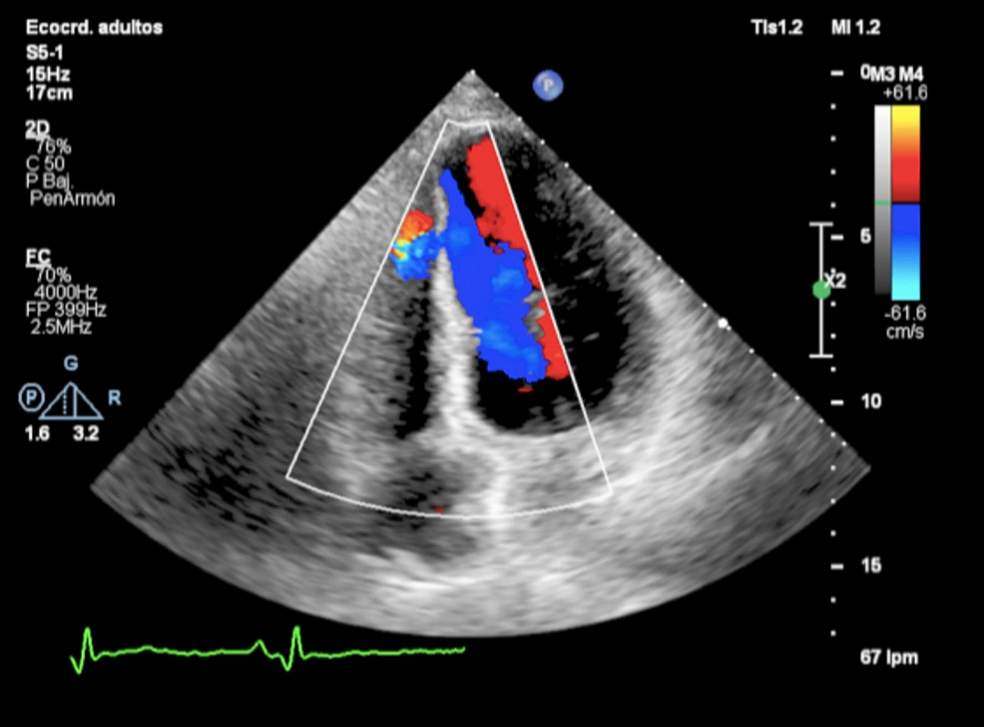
Ventricular septal defect observed by cardiac Doppler echo
